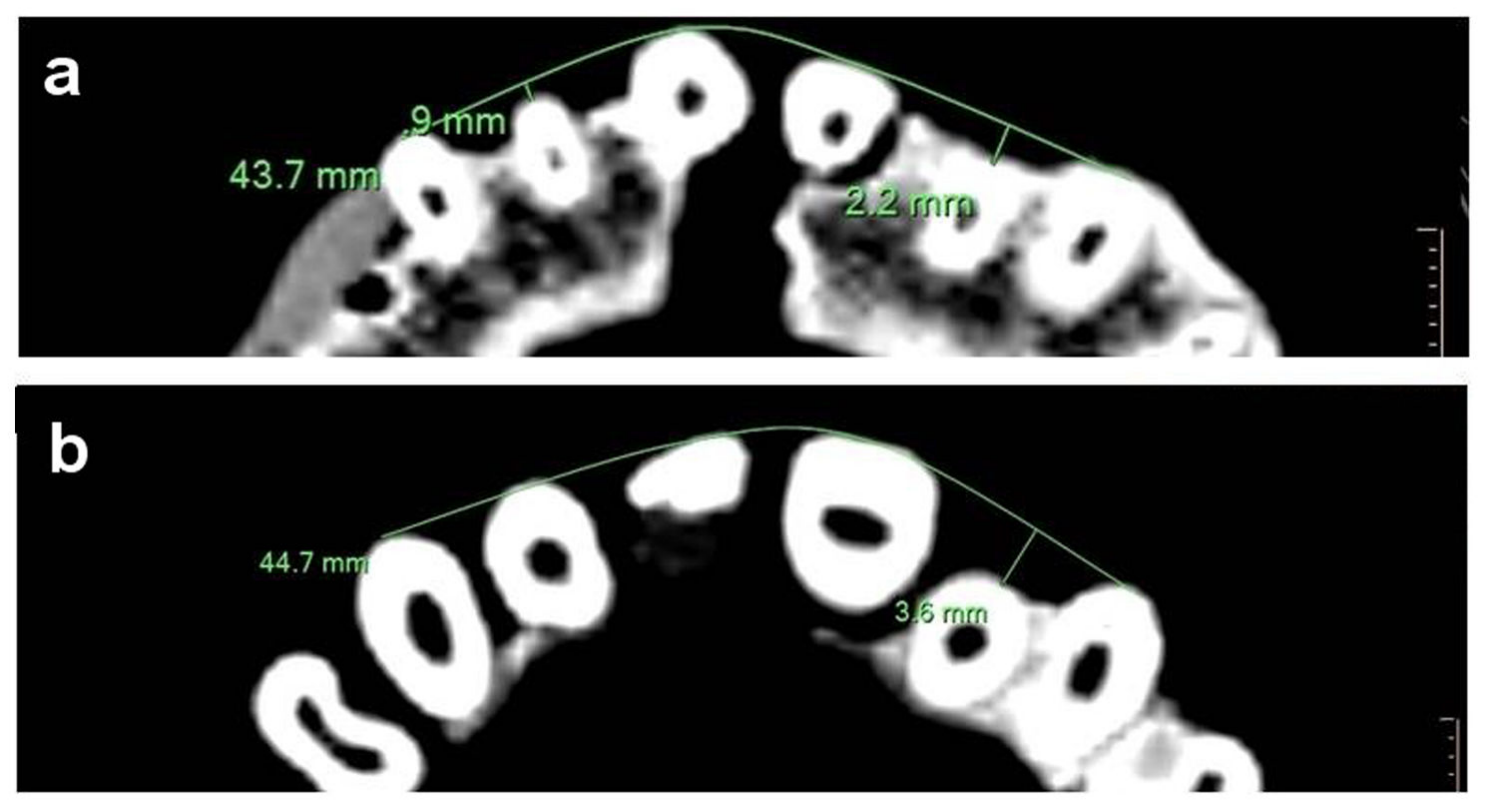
CT measurement of the position of the upper left lateral incisor. Measurements are shown in the apical (a) and gingival (b) segments.
